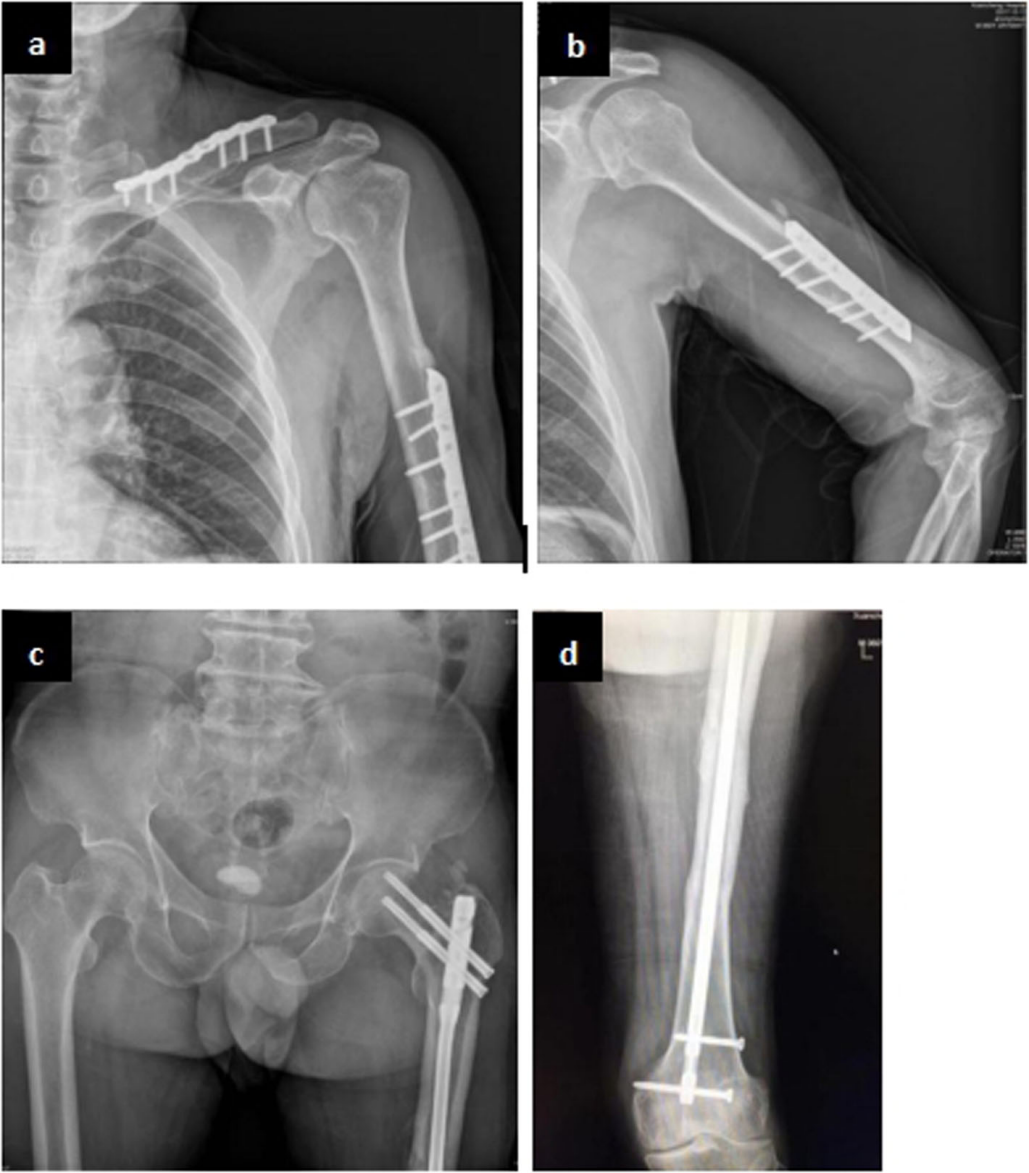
Postoperative radiograph of the left clavicle (a), humeral shaft (b), femoral neck and femoral shaft (c, d) (3 years after surgical)
